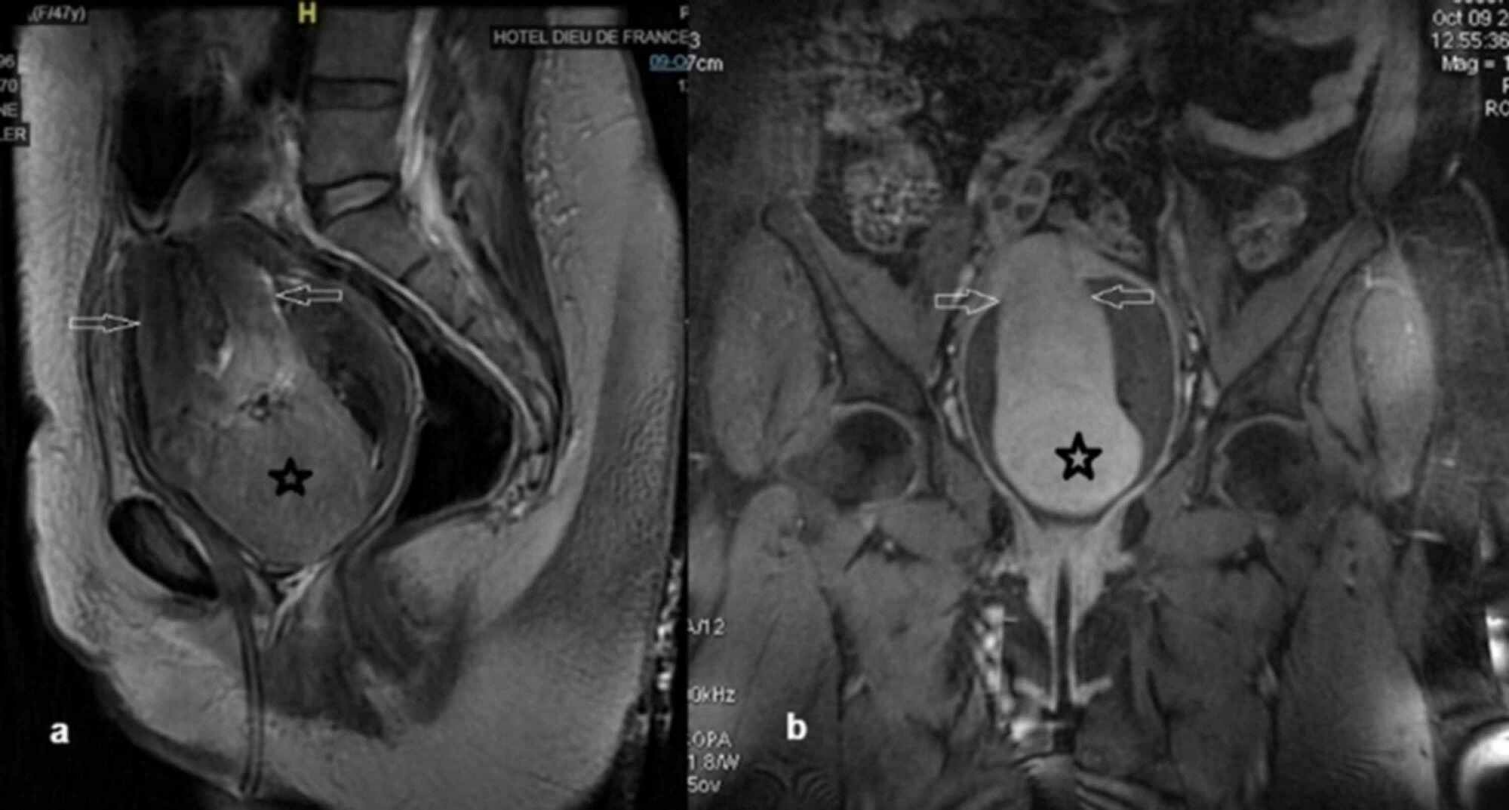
MRI characteristics of the uterine inversion In a: T2-sagittal view, In b: coronal view. The two arrows depict the dumbbell appearance. The myoma is depicted by a *.
